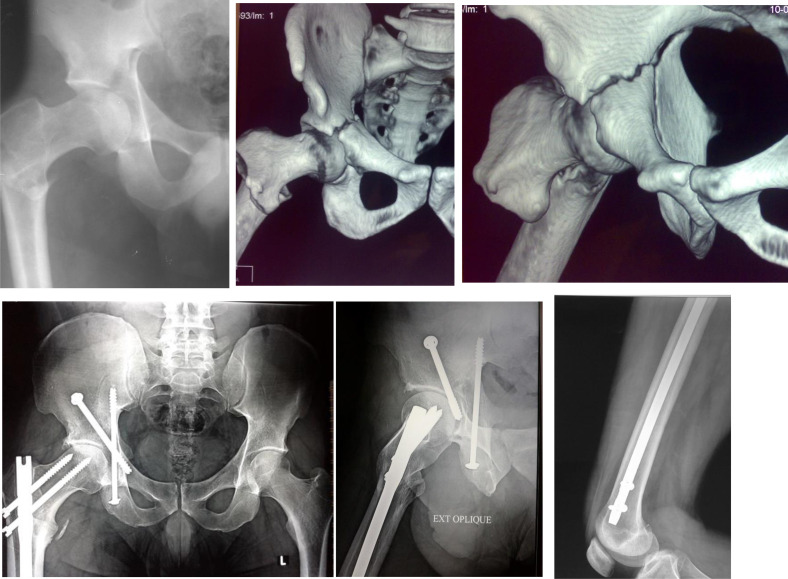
Case example showing ipsilateral acetabular fracture and proximal femoral fracture. Top row: Preoperative X-ray and 3D- reconstruction CT scan. Bottom row: X-rays at 7 years postoperative.
